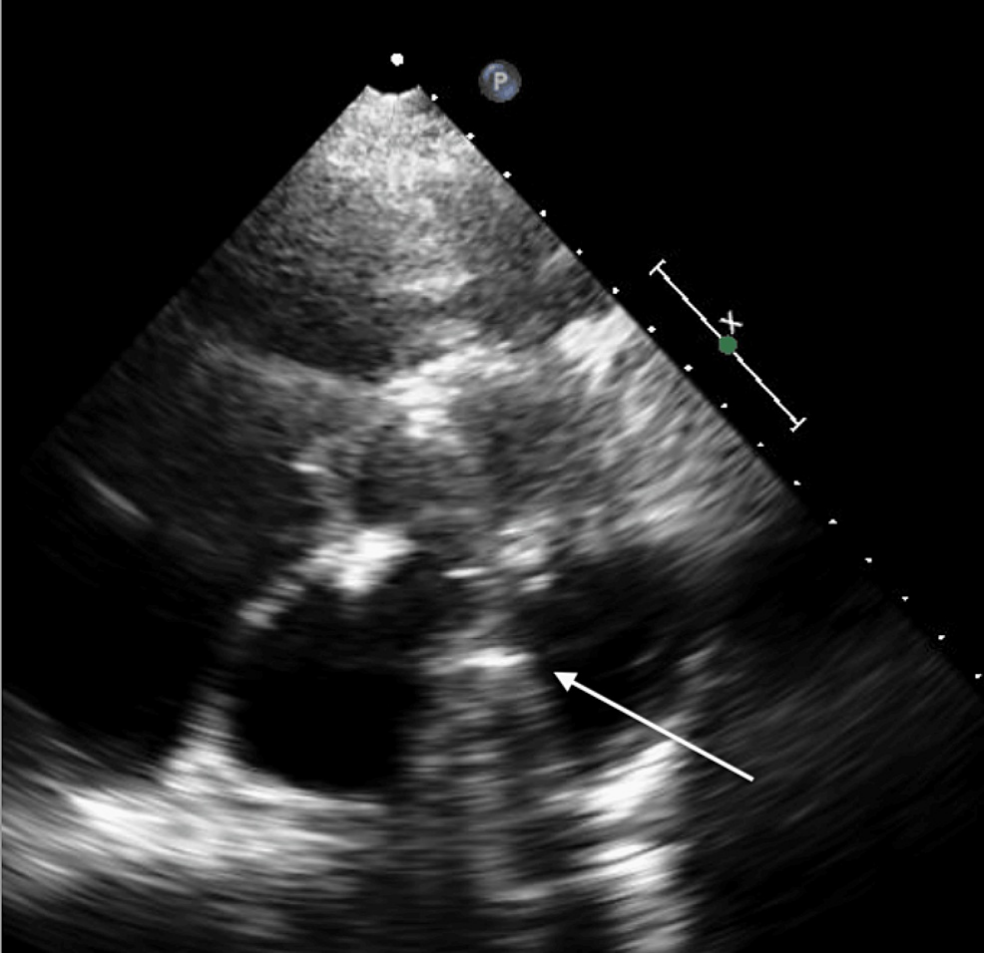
A short parasternal view TTE showing an echo dense mass (white arrow) representing the IABP in the LV TTE: trans-thoracic echocardiogram; IABP: intra-aortic balloon pump; LV: left ventricular
